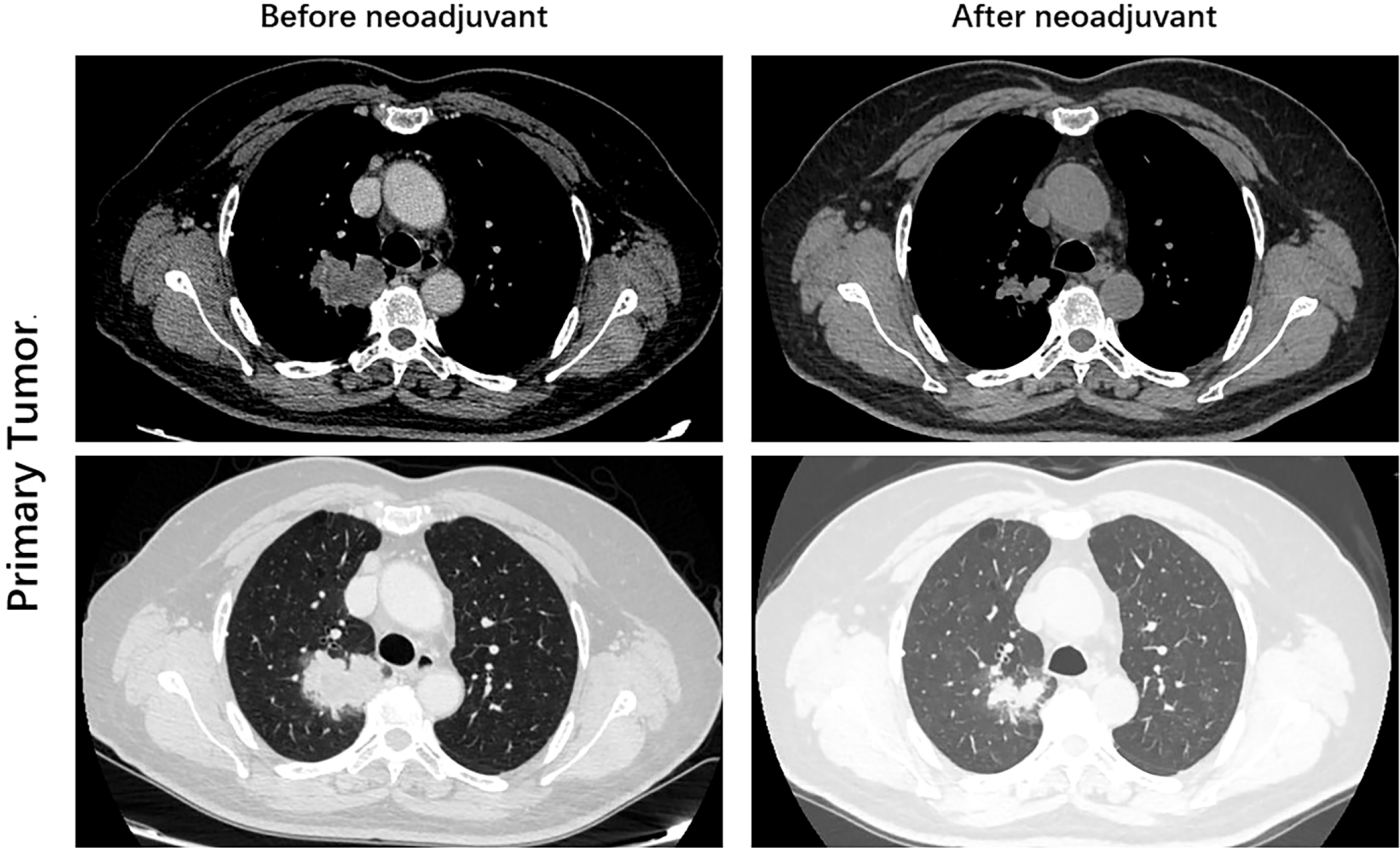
Images during neoadjuvant savolitinib treatment. Enhanced chest CT images of right lung adenocarcinoma before and after the neoadjuvant therapy.
